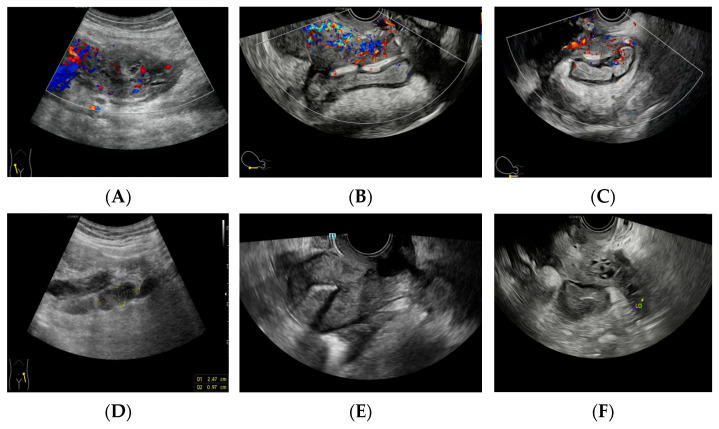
The preoperative ultrasound detection of pelvic mass: (A) ultrasound detection of a mass of about 8.8 × 4.4 × 4.8 cm in the right adnexa, with abundant blood flow signals, RI: 0.65. (B) The mass is not clearly demarcated from the rectum, sigmoid colon, and sacroiliac ligament. (C) Ultrasound shows thickening and decreased echo of the intestinal wall behind the uterus, with abundant blood flow. (D) Multiple enlarged lymph nodes in the bilateral iliac fossa. (E) A copper-bearing intrauterine contraceptive device, in the normal position. (F) The size and shape of the left ovary are acceptable.
